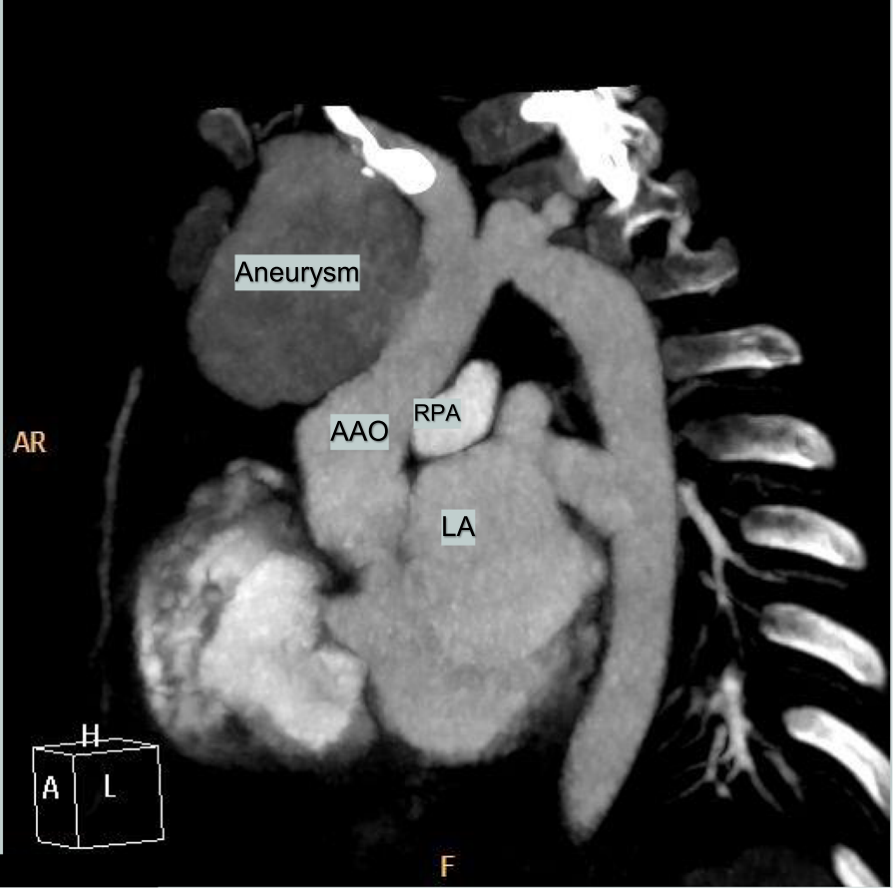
Computed tomography angiogram which shows a pseudoaneurysm arising from the anterior wall of the ascending aorta. Dimensions were 4.5 × 4.0 × 3.5 cm with a 10–11 mm neck. The aneurysm extended to the right and superiorly causing rightward deviation of the superior vena cava and innominate vein *AAO* ascending aorta, *LA* left atrium, *RPA* right pulmonary artery
